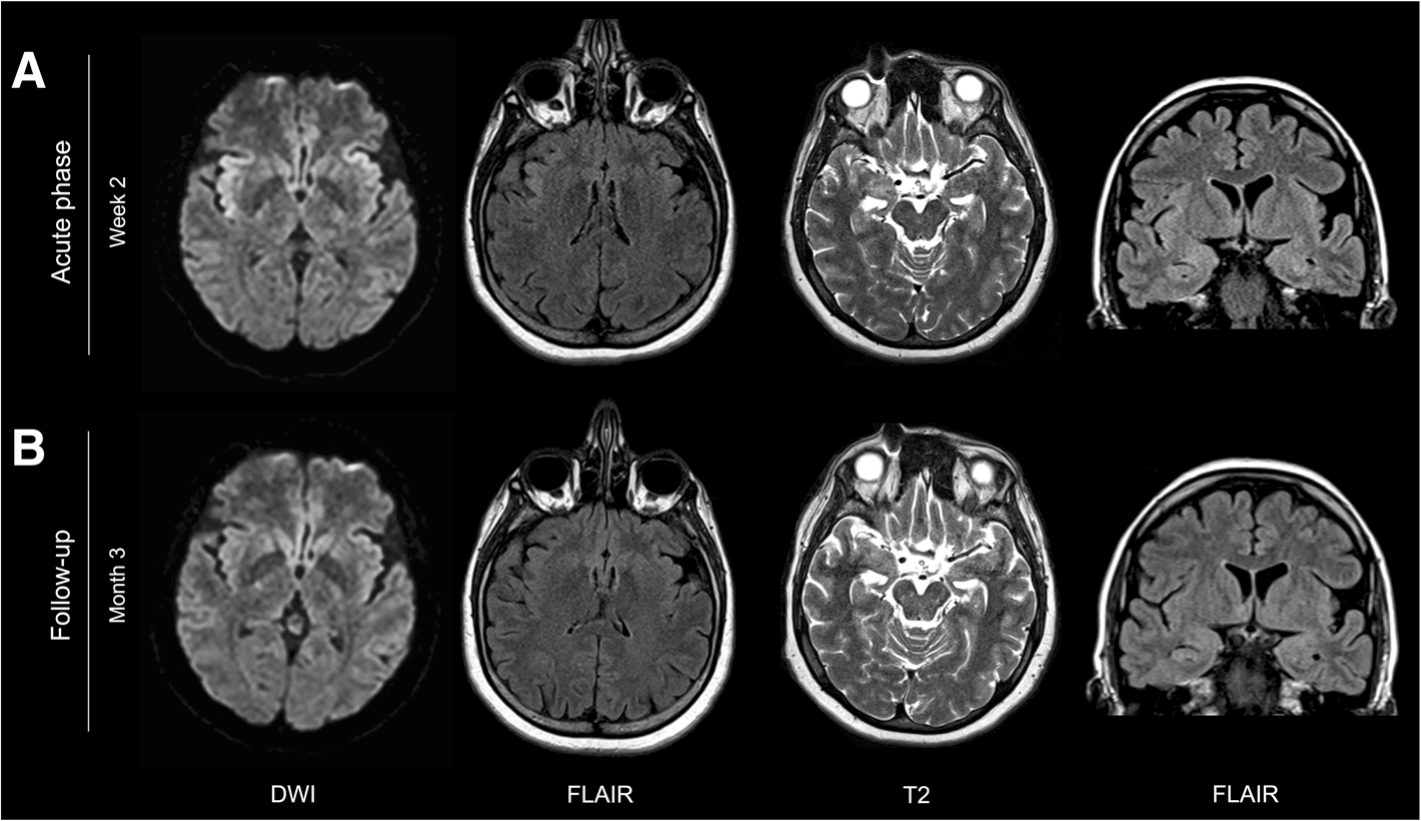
Insular and hippocampal MRI abnormalities. a MRI during acute phase of the disease (2 weeks after onset) shows diffusion restriction on diffusion-weighted images (DWI), hyperintensity on fluid-attenuated inversion recovery (FLAIR) images bilaterally, right-accentuated in the insular cortex as well as slight hyperintensity on T2-weighted and FLAIR images in both hippocampi. b After 3 months with substantial clinical improvement bi-insular diffusion restriction and FLAIR abnormalities have disappeared; hippocampal T2/ FLAIR hyperintensities are still visible
